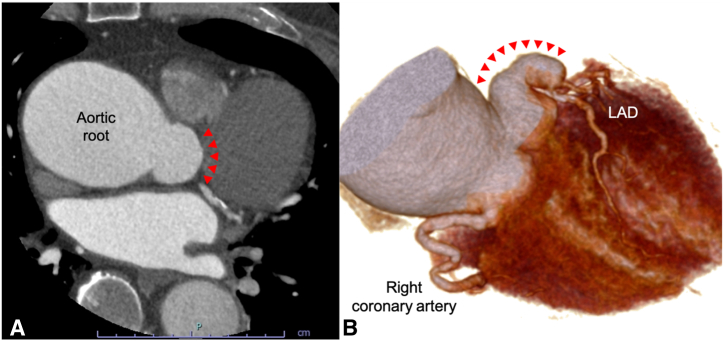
Preoperative computed tomography showing annuloaortic ectasia (A) and a left main coronary artery aneurysm extending to the bifurcation (B). *LAD*, Left anterior descending artery. The *arrowhead* symbol represent the LMCA aneurysm.
